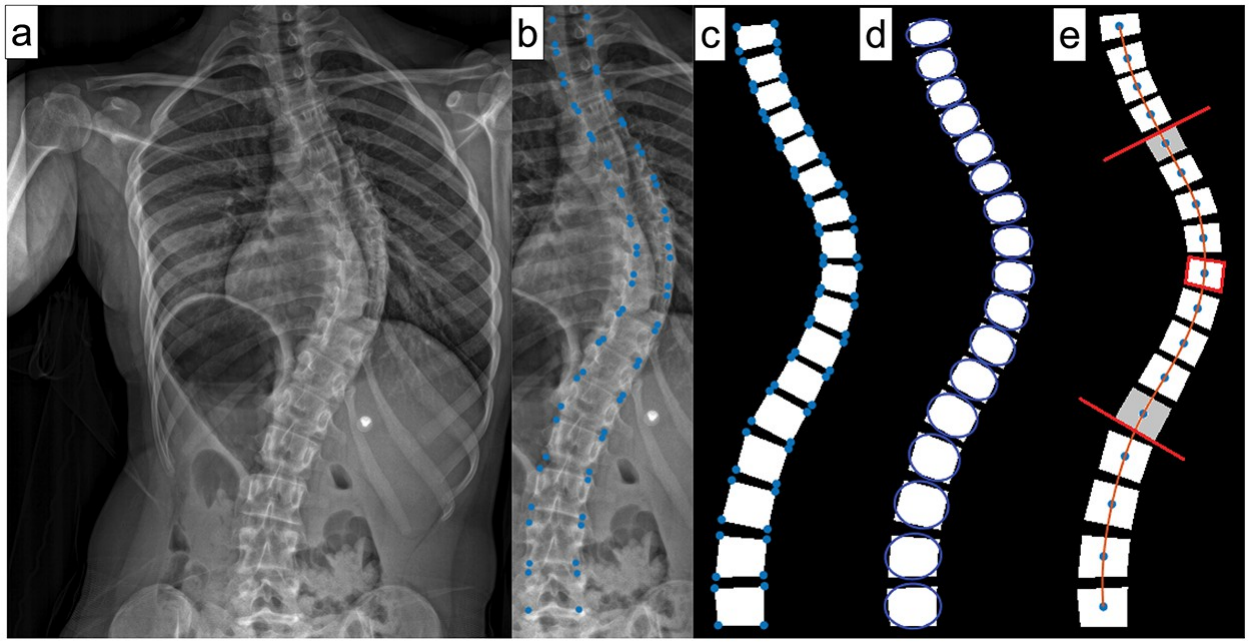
Steps of Cobb angle measurement from frontal radiographs. a. Plain frontal X-ray b. Four LMPs were selected per vertebra c. Polygon fit through each vertebra vertices (LMPs) d. Best fit ellipse through each polygon to calculate the orientation of each vertebra as the angle between the major axis of the ellipse and the horizontal e. A cubic spline was fit through the centroids of the vertebrae, the most tilted vertebrae above and below the apical level were identified, and the Cobb angle was calculated between these vertebrae.
